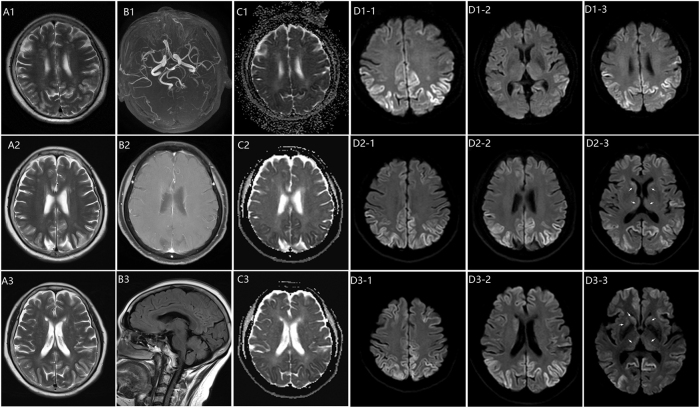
Serial brain MRI progression in prion disease. Images acquired on admission day 1 (top row), day 3 (middle row), and day 7 post-glucocorticoid (bottom row), across T2-weighted, MRA, ADC, and DWI sequences (left to right). Key DWI findings: day 1 – cortical ribbon sign with hyperintensity in bilateral temporoparietal and occipital cortices; day 3 – development of hockey stick sign in bilateral caudate heads and medial thalami (arrows); day 7 – new hyperintensity in the right globus pallidus (arrow) and more extensive cortical and deep gray matter involvement.
